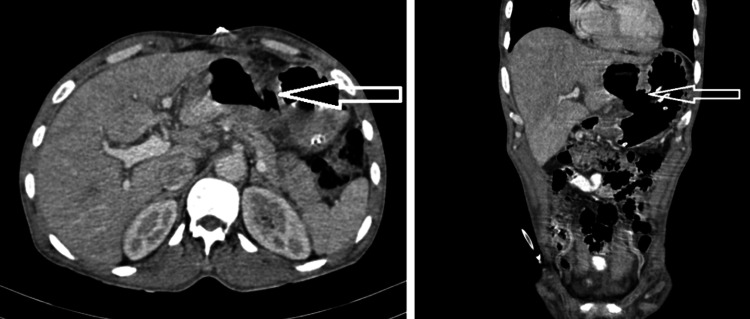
Axial and coronal contrast-enhanced CT shows a well-defined saccular outpouching noted at the lesser curvature stomach (at the body) filled with oral contrast and seen communicating (neck size: 2.8 cm) with the gastric cavity.
